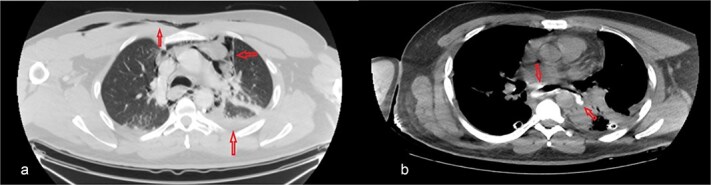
Chest CT scan: (a) with IV contrast, extensive pneumomediastinum as well as extensive cervical and anterior chest subcutaneous surgical emphysema with mild bilateral pleural effusion. (b) IV and oral contrast: Evidence contrast leakage arising from the left posterolateral aspect of mid esophagus associated with mild left loculated pleural effusion and mild pneumomediastinum.
